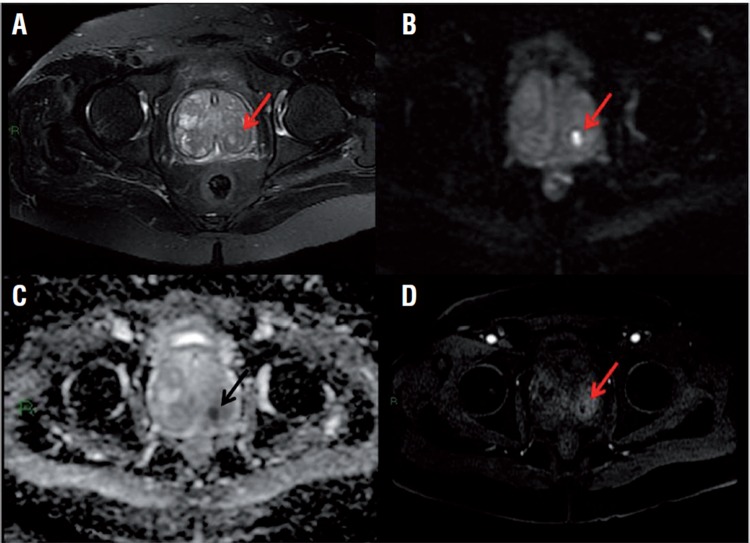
Prostate MRI showing a small nodular area (arrows) in the left posterior mid-third of the transition zone. This lesion shows liquid content on T2-weighted images (A), and marked diffusion restriction (B), with corresponding ADC map (C) and intense peripheral enhancement (D), findings suggestive of an abscess.
